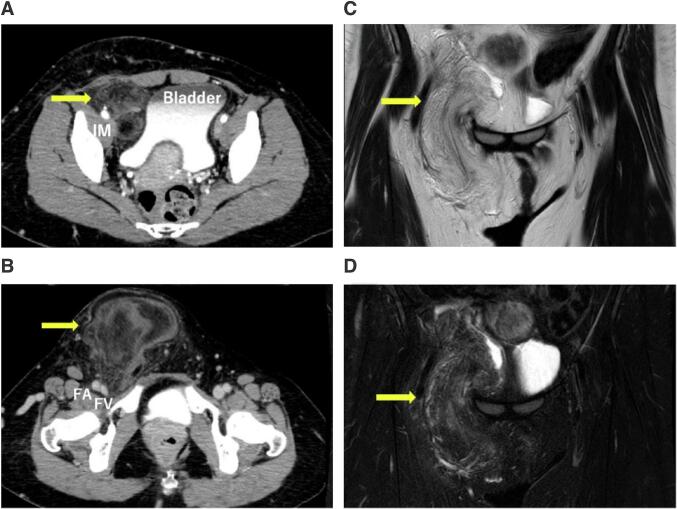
On the CT scan, although the tumor extended from the retroperitoneum into the inguinal region, there was no evidence of bladder invasion (A). In the inguinal region, the tumor was in contact with the femoral artery and vein (B). The tumor had a high signal intensity On T2-weighted MRI imaging (C), and a low signal intensity on fat-suppression MRI imaging (D). IM: iliopsoas muscle, FA: femoral artery, FV: femoral vein.
